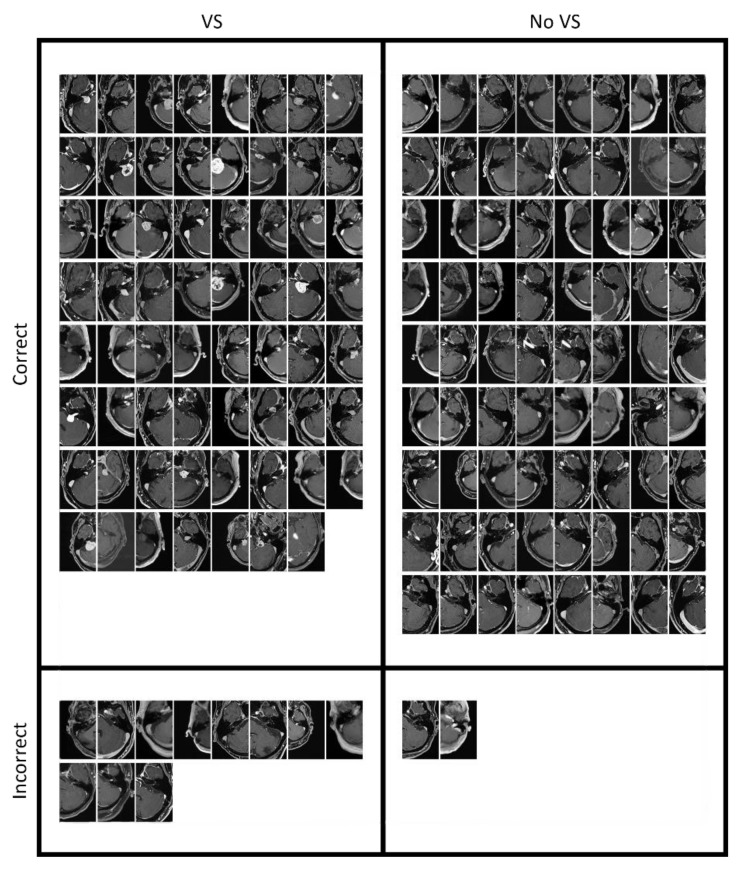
All MRI slices of the external validation data set with the T1c sequence. The images are sorted based on whether they contained a VS and whether they were correctly classified.
